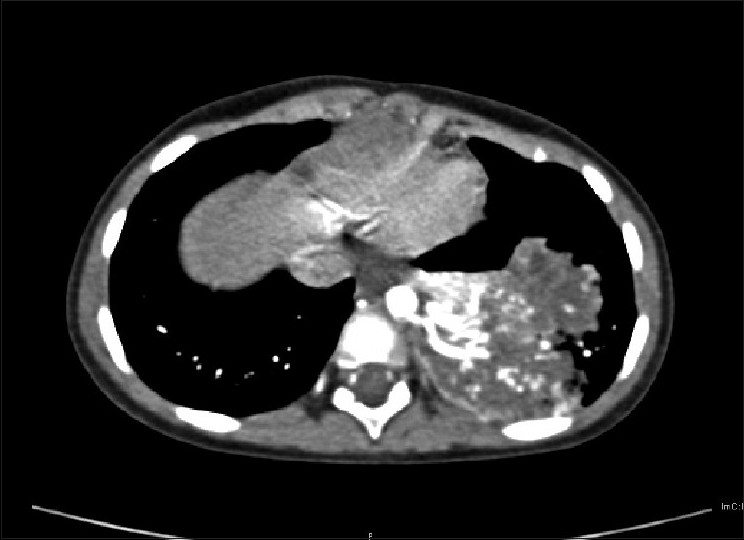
Axial CT angiographic image showing anomalous vessels arising from the descending aorta and supplying the enhancing left lower lobe sequestered lung segment
